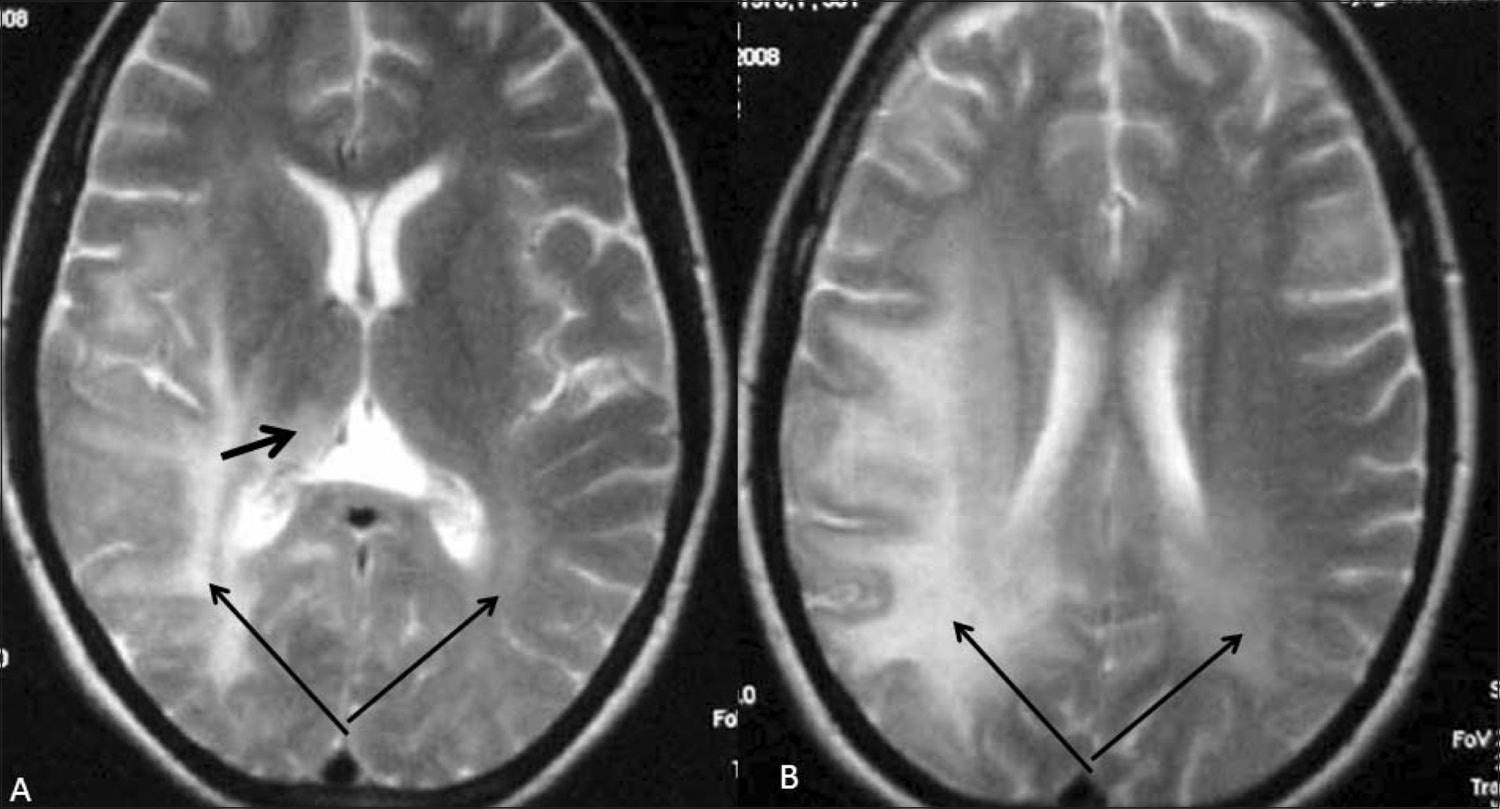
(A and B) Axial T2W MRI images of the brain show bilateral, asymmetric, confluent white matter hyperintensities involving the posterior aspect of the brain, extending from the subcortical white matter (long arrows) to the periventricular white matter. Also noted are the involvement of the thalamus (short arrow in A) and the absence of mass effect or atrophy
